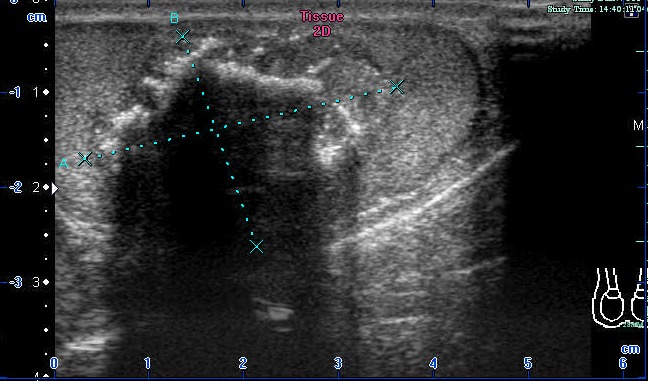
Scrotal ultrasound revealed a roundish, well-demarcated, hypoechoic mass with calcifications in the left testicle.
